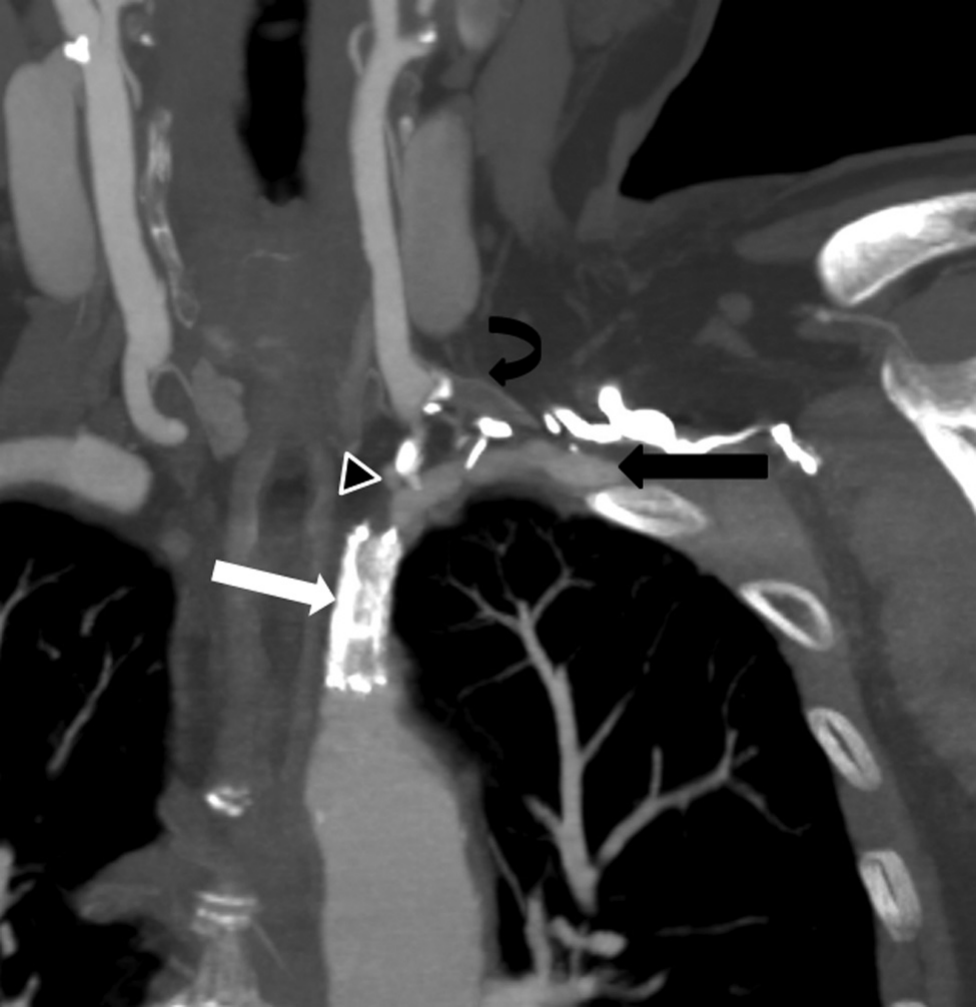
Curved reformat computed tomography angiography with maximal intensity projections at 8 mm demonstrates occluded left subclavian artery stent (white arrow) and occluded left common carotid artery to subclavian artery bypass graft (curved black arrow). The left subclavian artery (black arrow) is predominately supplied by retrograde flow as seen at the left vertebral artery origin (black arrowhead).
